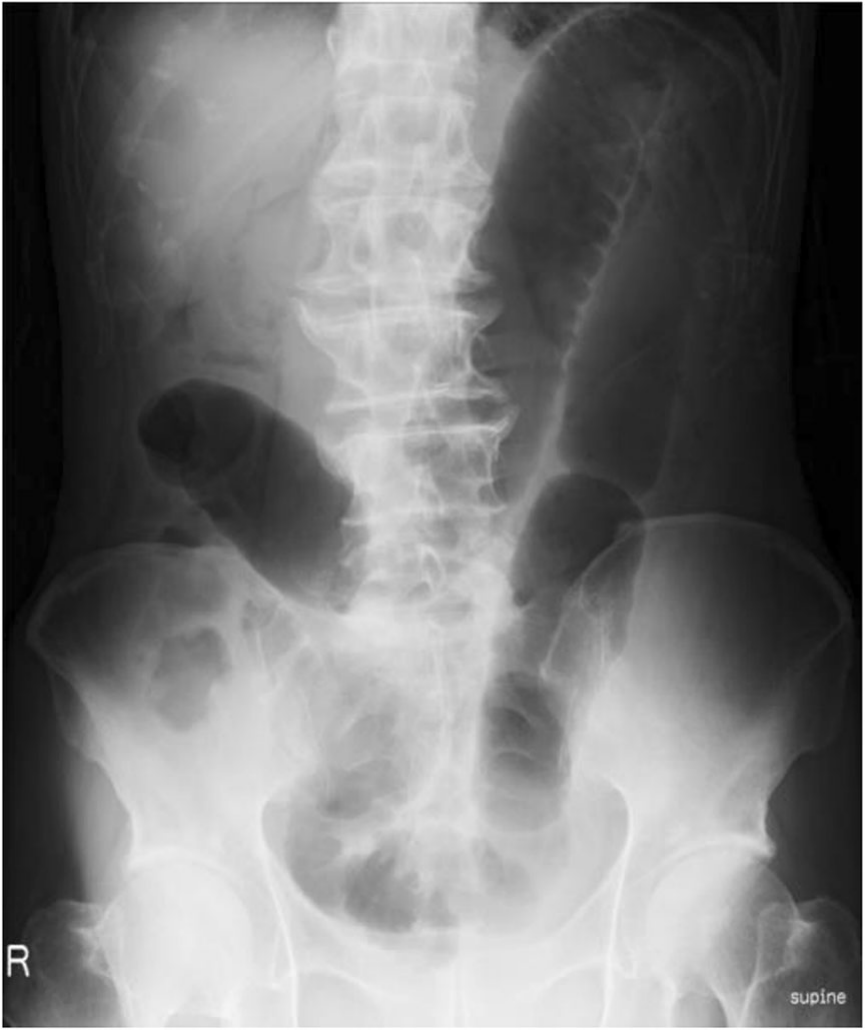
In X-ray on the 6th postoperative day, a gas was seen throughout the dilated colon
